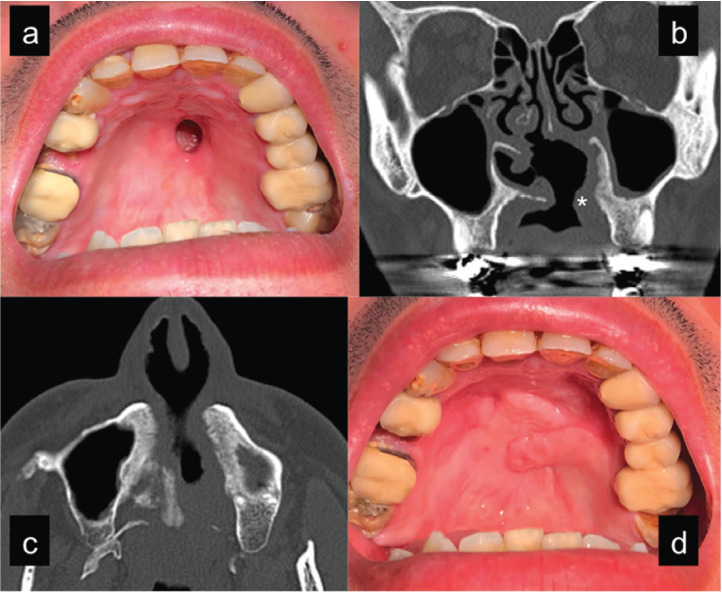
Patient with a 1 cm perforation in the anterior hard palate (a) that can be appreciated (mark) in a coronal CT scan (b). Complete bone loss in the left palatal side in an axial view (c). For this reason the inverted flaps for the nasal lining were partial thickness and the left rotational flap was harvested from the alveolar palatal wall, where underlying bone was present. Postoperative view (6 months) (d) after performing a double opposite pediculated palatal flap.
